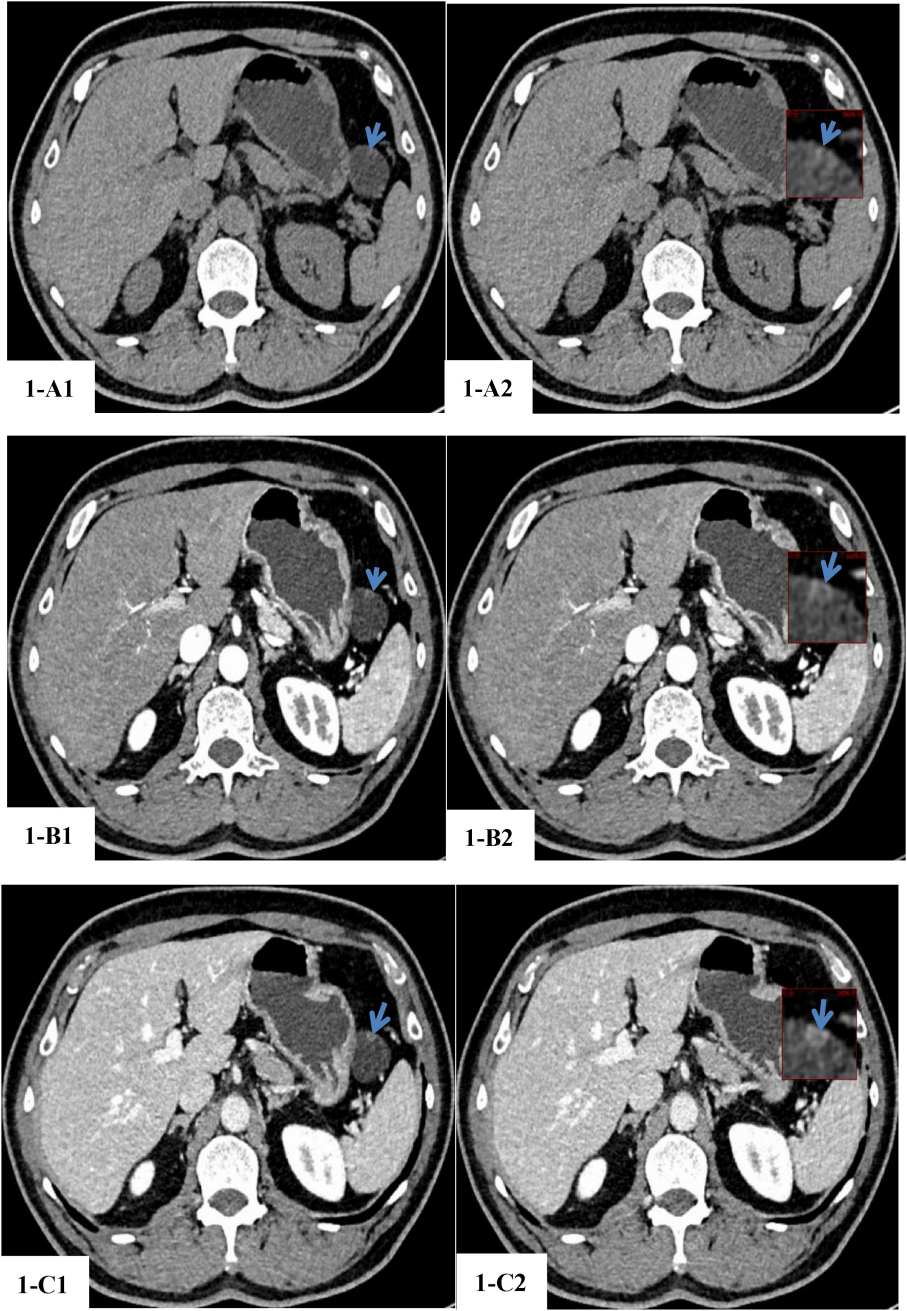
CT images of a space-occupying lesion in the tail of the pancreas. (1-A1) High-resolution non-contrast CT scan with thin transverse sections: the lesion is located in the pancreatic tail, appears ovoid, demonstrates hypodensity (CT value: −12 HU), has maximum dimensions of approximately 3.08 cm × 2.82 cm × 2.82 cm, and corresponds to the region indicated by the blue arrow. (1-A2) High-resolution non-contrast CT scan with thin transverse sections: upon magnification, a high-density nodular opacity with a CT value of 17 HU is visualized within the cyst wall, and corresponds to the region indicated by the blue arrow. (1-B1) Thin-section transverse CT scan of the arterial phase following contrast enhancement: the CT value of the hypodense region within the tumor lesion is approximately −18 HU, and corresponds to the region indicated by the blue arrow. (1-B2) Thin-section transverse CT scan of the arterial phase following contrast enhancement: upon magnification, a mural nodule with a diameter of about 0.6 cm was visible at the anterior margin of the lesion, which showed significant enhancement after enhanced scanning, and the CT value of the region is approximately 26 HU, and corresponds to the region indicated by the blue arrow. (1-C1) Thin-section transverse CT scan of the venous phase following contrast enhancement: the CT value of the hypodense region within the tumor lesion is approximately −21 HU, and corresponds to the region indicated by the blue arrow. (1-C2) Thin-section transverse CT scan of the venous phase following contrast enhancement: upon magnification, the CT value of the mural nodule of the tumor cyst wall is 44 HU, and corresponds to the region indicated by the blue arrow.
